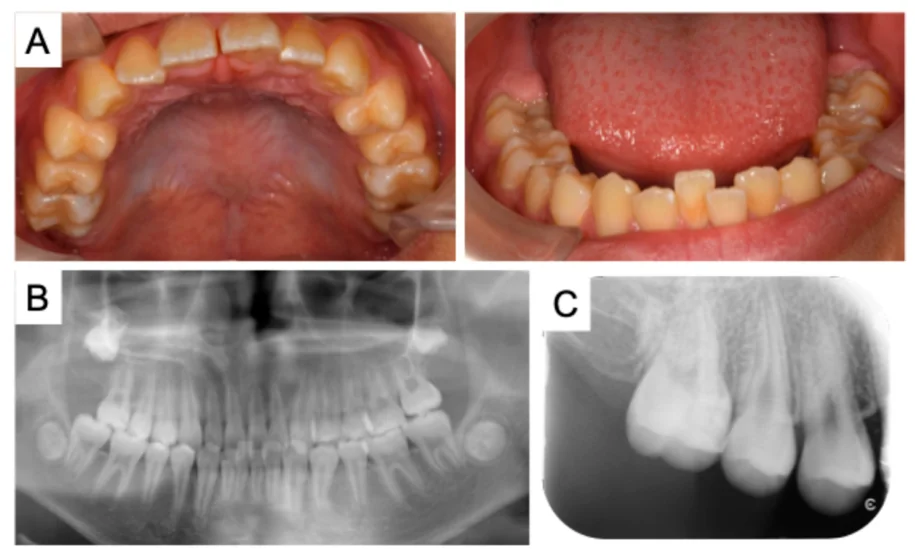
Intraoral and panoramic examinations at the initial visit. An 11-years-and-11-months-old boy was referred to our hospital with a chief complaint of dental caries. A routine school dental checkup had detected the caries. He visited a private dental clinic but was unable to receive treatment because of uncooperative behavior and a pronounced gag reflex. The medical and family histories were otherwise unremarkable, except for intellectual disability. Twenty-seven permanent teeth, except for the maxillary right second molar, had erupted into the oral cavity (A). Panoramic examination revealed the presence of the maxillary right second molar, with a well-defined radiolucency surrounding the crown (B). Root formation was slightly delayed compared with the contralateral tooth; however, eruption appeared markedly delayed. A periapical radiograph could not provide adequate evaluation because of the gag reflex (C). Dental caries were identified in multiple molars, and caries treatment was prioritized. A detailed examination of the maxillary second molars was scheduled subsequently. Caries treatment was performed under physical restraint with parental consent.
